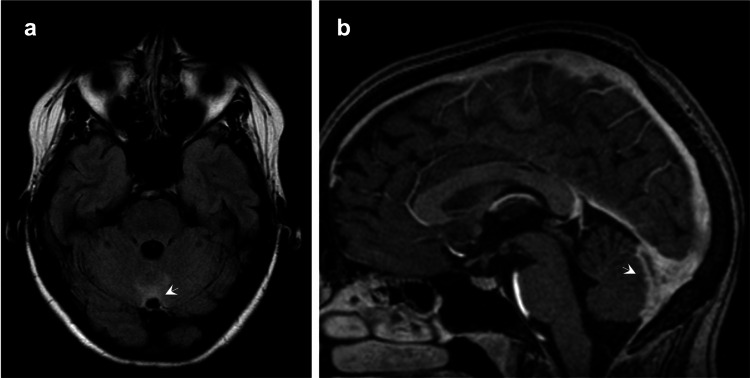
Five weeks after the initial cerebral MRI, (a) T2WI-FLAIR shows a reduction in edema in the vermis (arrow). (b) Contrast-enhanced T1WI reveals a decrease in pachymeningeal enhancement (arrow). T2WI: T2-weighted imaging; FLAIR: fluid-attenuated inversion recovery; T1WI: T1-weighted imaging.
